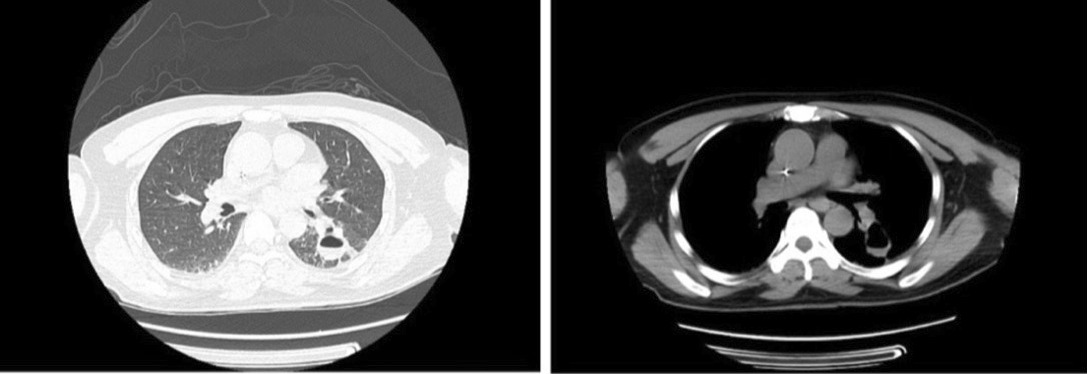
Lung CT scan revealed cavernous lesions in the lower lobe of the left lung and a few fibrous foci in the right lung's middle lobe, as well as a minor amount of pleural effusion
